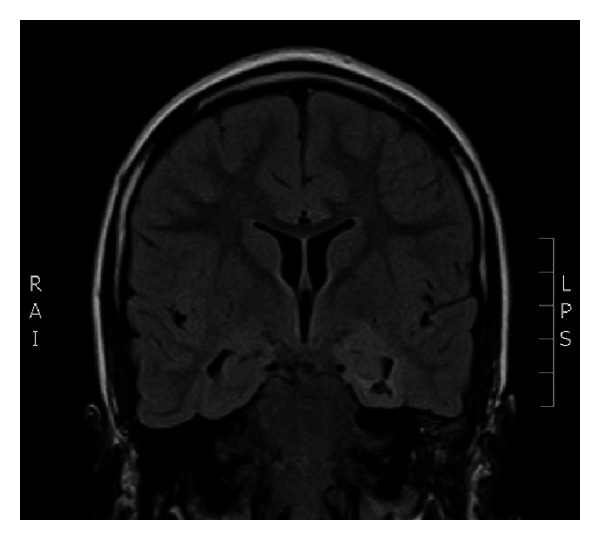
Coronal FLAIR MRI scan demonstrating the left mesial temporal lesion.
